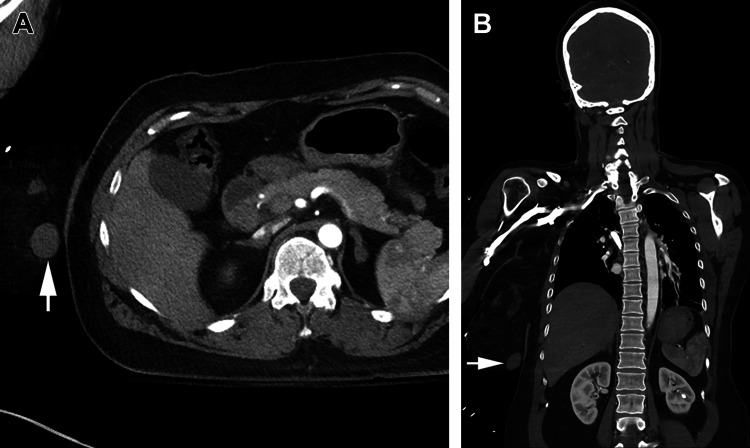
Incidental breast mass Axial (A) and coronal (B) CT images showing an incidental right breast mass (arrows) identified on WBCT. Original image available from analysis by Gunn et. al published in Radiologic Clinics of North America [7]. CT, computed tomography; WBCT, whole-body computed tomography
